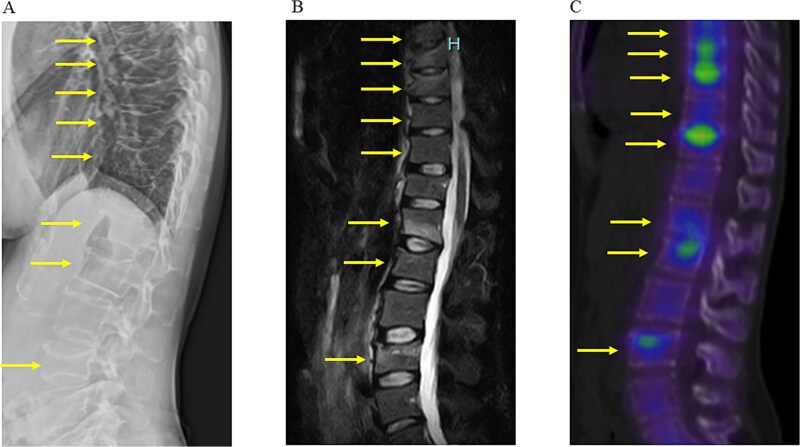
Pretreatment LS imaging. (A) Lateral radiograph of the vertebrae. (B) Lateral short tau inversion recovery (STIR) magnetic resonance imaging of the vertebrae. (C) ^99m^Tc-HMDP bone scan of the vertebrae. Arrow indicates the vertebral fracture.
